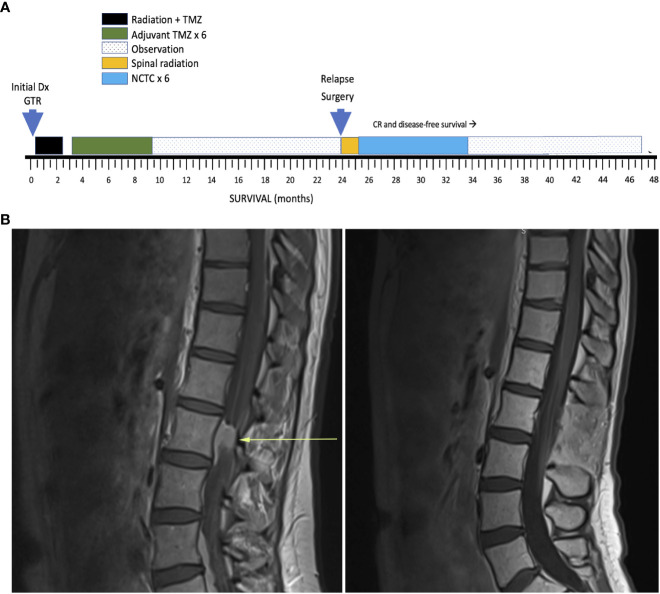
(A) Timeline of key events. GTR, Gross total resection; NTCT, network-targeting combination therapy (see text for details); CR, Complete remission. (B) Lumbar spine MRI T1 sagittal LEFT: at relapse and RIGHT: 10 months after NTCT during remission.
